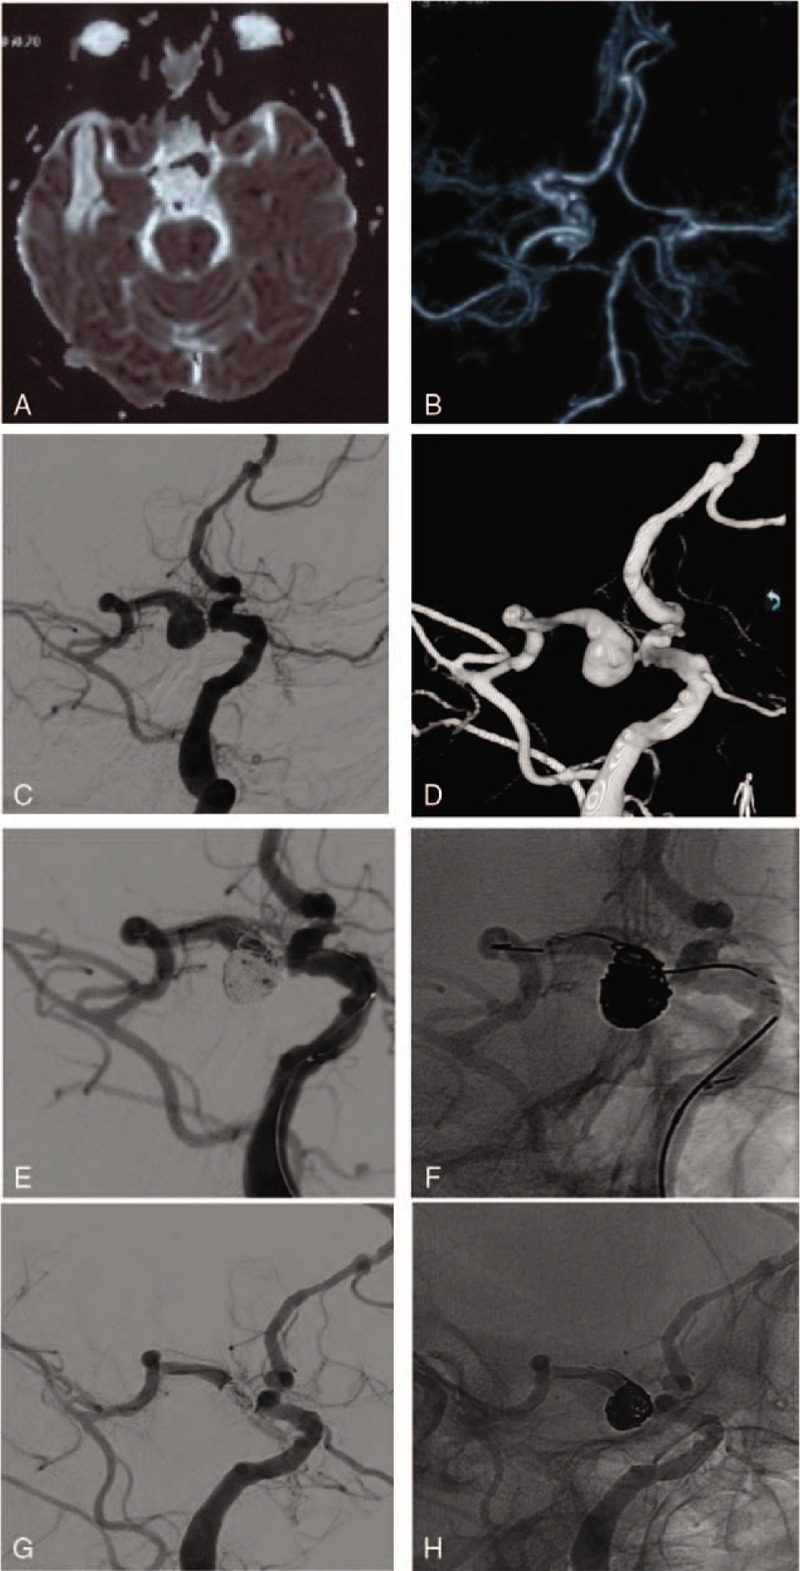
Images of the patient 3. A: Head MRI showing blood flow void beside the brain stem; B: Head CTA showing the ectasia of the left PComA; C: Left ICA injection angiograms showing a ectatic and tortuous PComA and PCA with 1 aneurysm located in PComA; D: Left ICA 3D injection angiograms; E: Embolization of the aneurysm by coils assisted by Stent (silhouette image); F: Embolization of the aneurysm by coils assisted by Stent; G: FU 3 months later, the aneurysm was disappear and the vascular morphology was clear (silhouette image); H: FU 3 mo later, the aneurysm was disappear and the vascular morphology was clear.
